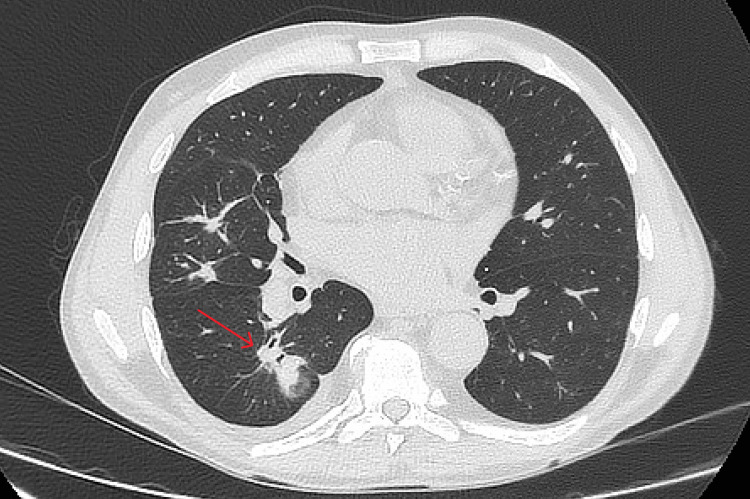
Follow-up chest computed tomography in March 2025 (red arrow indicating residual lung lesion in segment VI of the right lung).
